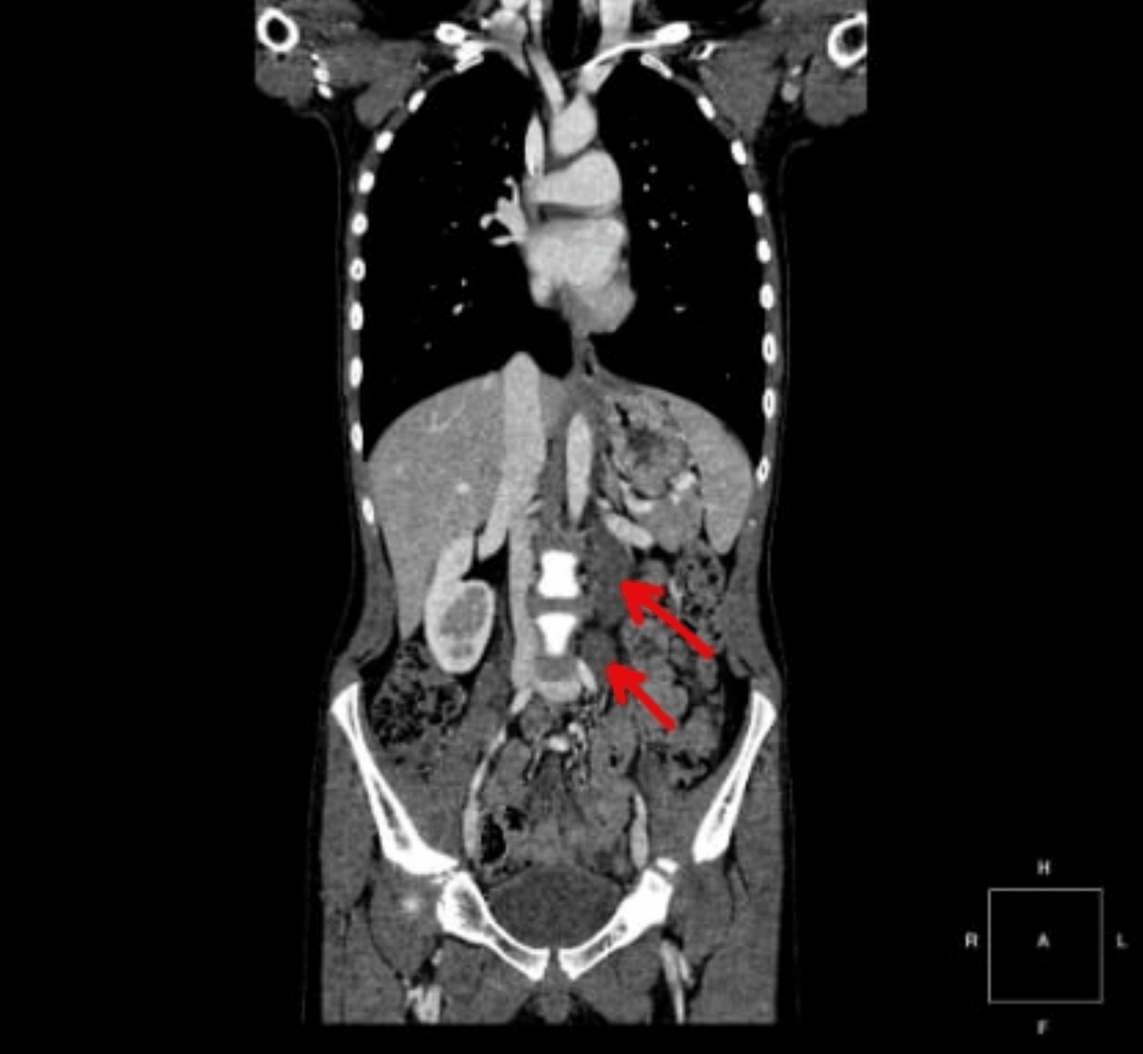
CT scan of the chest and abdomen showing enlarged lymph nodes in the left retroperitoneal space along the left psoas muscle (red arrows).
